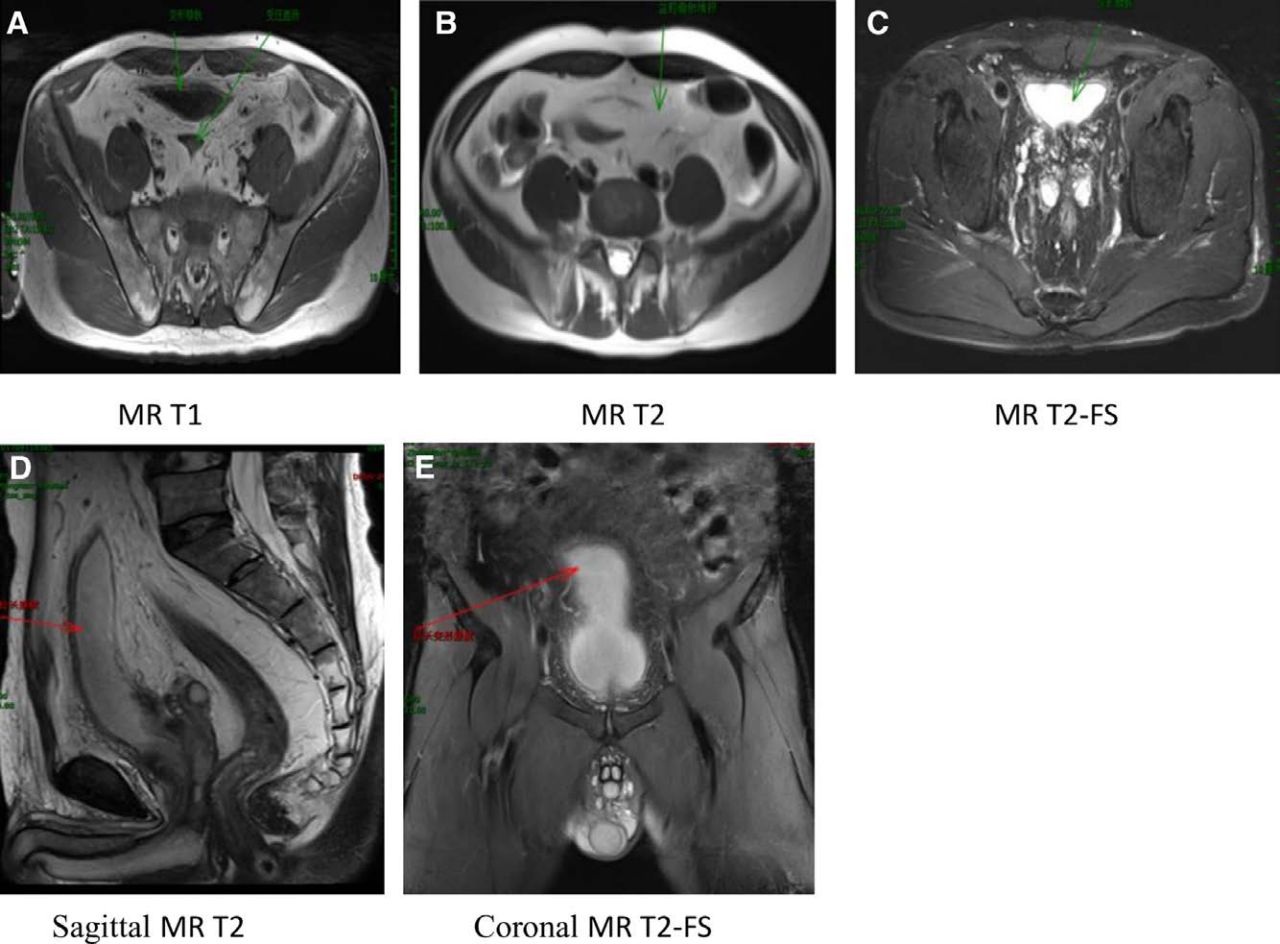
(A–E) Abdominopelvic MRI: (A–C) MRI shows a large amount of fat accumulation in the pelvic region, exhibiting short T1 and long T2 signals. The bladder and rectum are significantly compressed and deformed, with tortuous pelvic veins and notable changes in the position of the seminal vesicles. (D) The bladder base is visibly elevated, appearing long and slender like an eggplant or loofah, with deformation of the rectum, disappearance of the colonic tenia, and significant rigidity of the intestinal wall. (E) In the coronal fat-suppressed sequence, the deformed and inverted bladder, resembling a ping-pong paddle, can be observed. The bladder wall appears rough and thickened.
